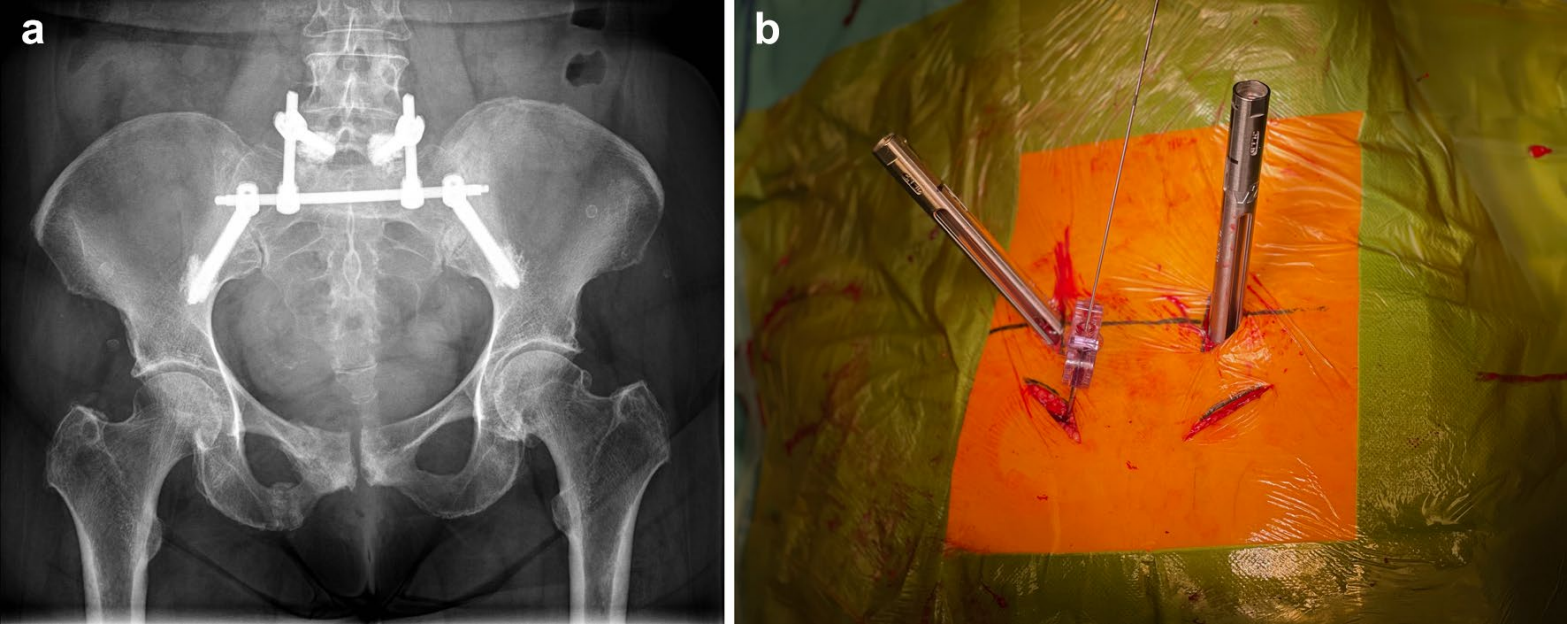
a and b X-ray pelvic overview (a) and intraoperative image (b) of a spinopelvic fixation
